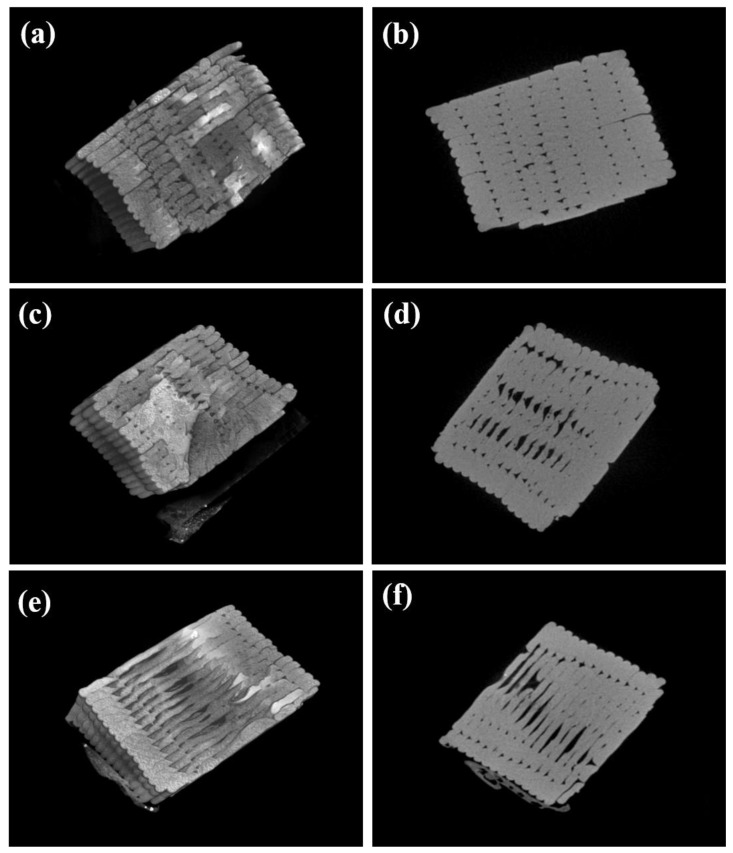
X-ray microtomography 3D images (a,c,e) and 2D slices (b,d,f) of tensile fractured 3D-printed TPI in (a,b) 0°, (c,d) 45°, and (e,f) 90° infill direction.
